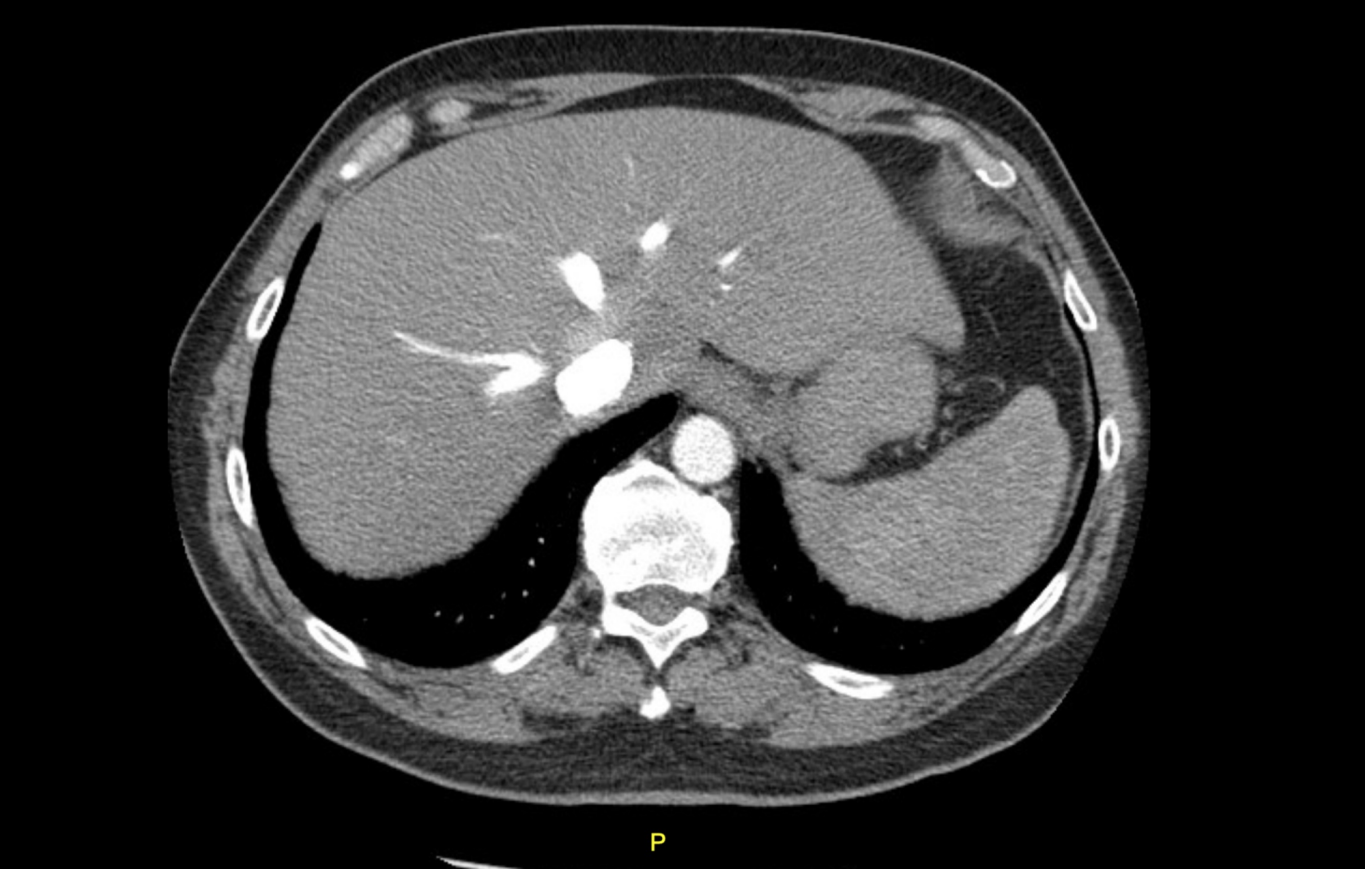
CT abdomen showing complete resolution of the abscesses after six weeks of treatment.
